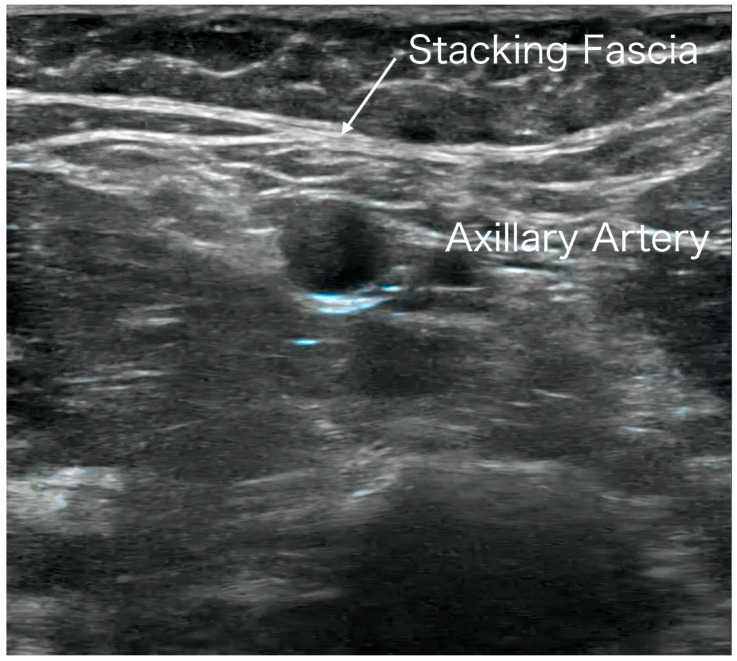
Ultrasound image of stacking fascia in the absence of an axillary arch. This figure shows stacking fascia in the axillary region of a patient without an axillary arch (Langer’s arch). A band-like hyperechoic stacking fascia can be seen superficial to the neurovascular bundle (arrow). This finding suggests that fascial memory may form at the same anatomical site through mechanical stress concentration even in the absence of accessory muscles. Release of this region is effective in conditions such as TOS and frozen shoulder (see Supplementary Video S2).
